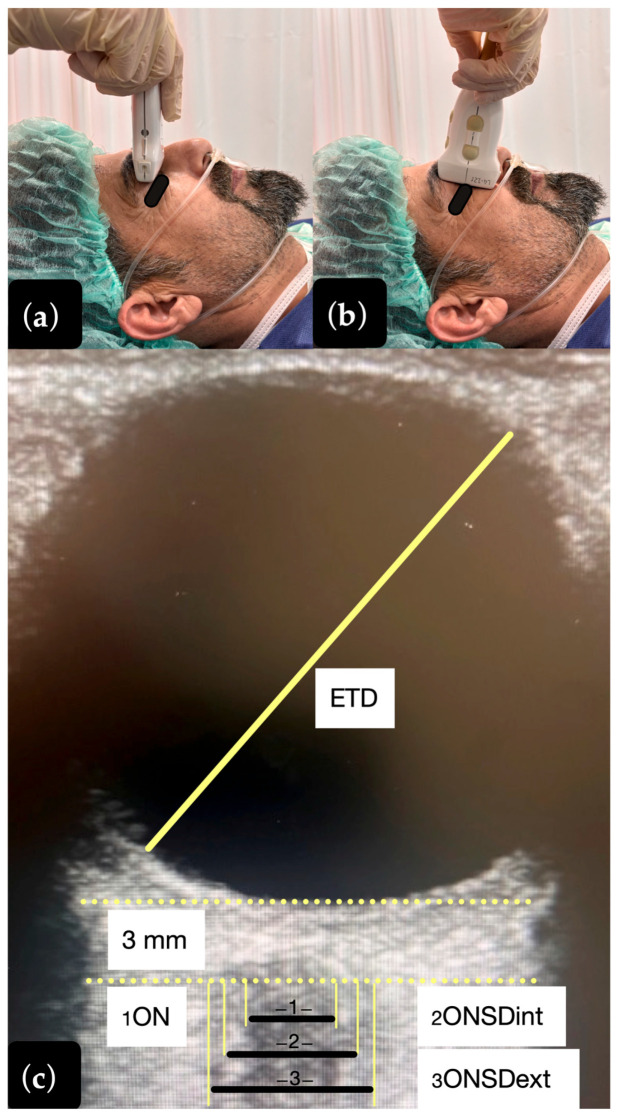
Ultrasound measurement. (a) Transverse and (b) sagittal probe positions. (c) B-mode image showing ETD, ONSDint and ONSDext. ONSD was measured at 3 mm posterior to the papilla.
